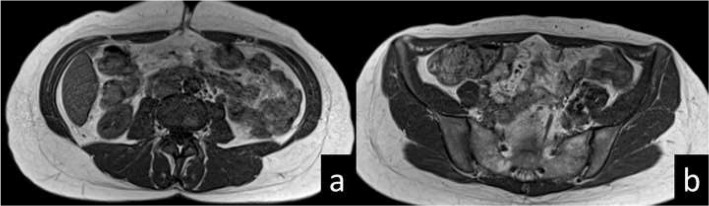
MRI of control. 40-year-old control. Transversal T1- weighted sequence in L3/L4- (a) and S1/S2-level (b) show normal muscles (score 0 and 1 on the Mercuri – score)
